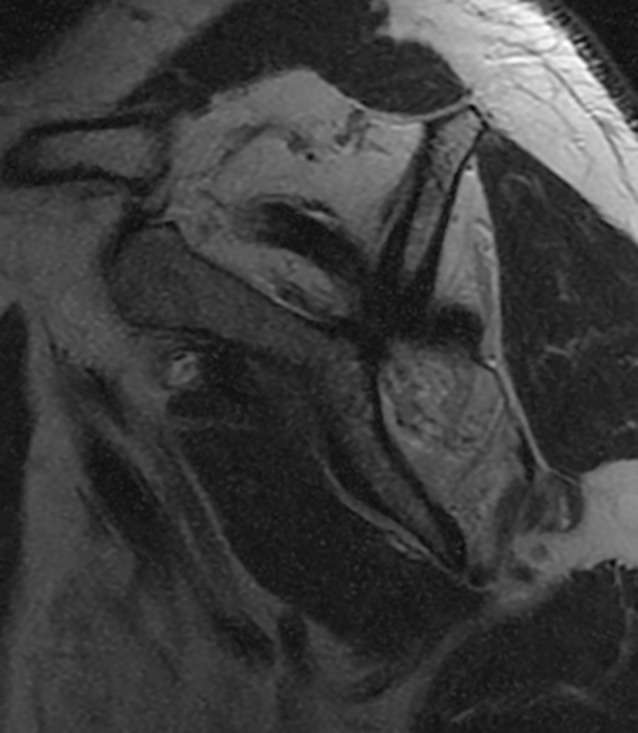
Sagittal T1-weighted magnetic resonance image demonstrating significant atrophy of the supraspinatus and infraspinatus tendons, representative of an irreparable rotator cuff tear
